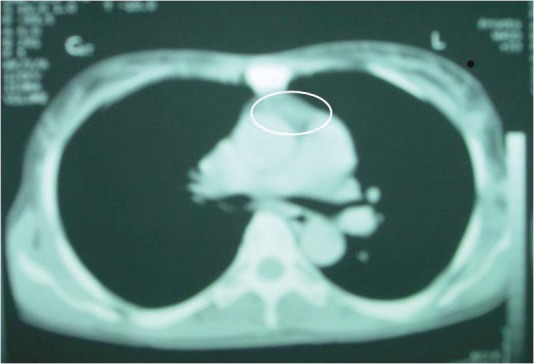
CT chest. Anterior mediastinal mass with minimal contrast enhancement.
